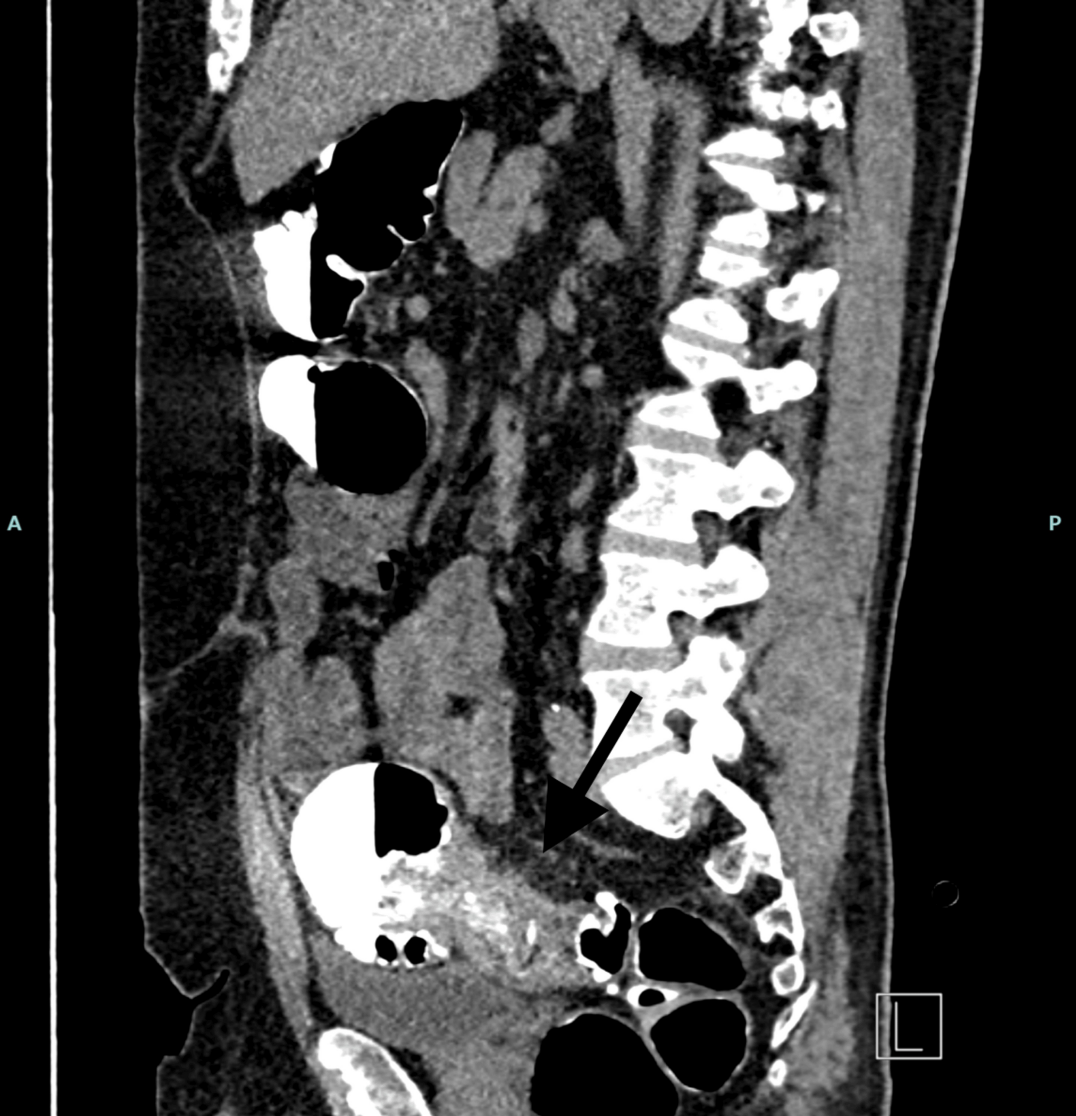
CT colonography revealing a circumferential shouldered lesion within the sigmoid colon, proximal to a segment of severe diverticular disease. The narrowing and mural thickening correspond to the fibrotic stricture identified intraoperatively, consistent with chronic diverticulitis rather than malignancy.
